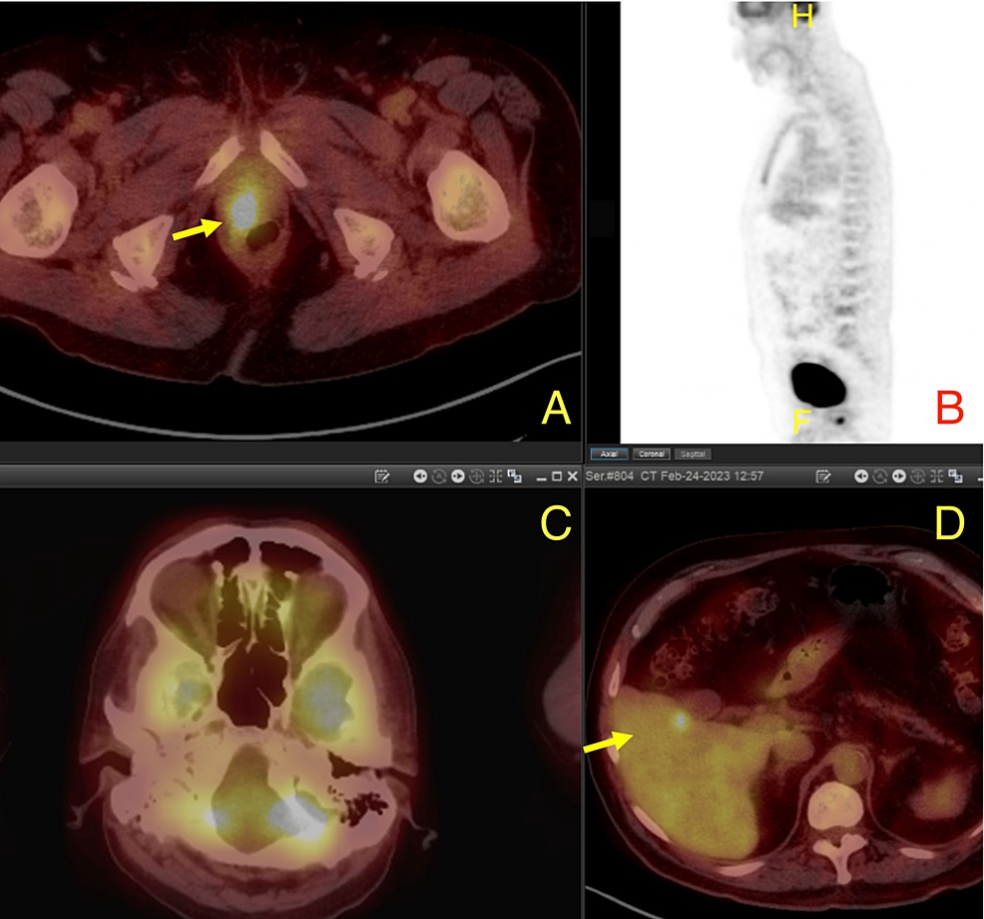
FDG-PET CT. (A) Increased uptake seen in apical-lateral prostate similar to area showing small cell neuroendocrine growth. (B) Skull not demonstrating enhanced growth near the area of orbital metastasis. (C) Spine not showing significant FDG enhancement. (D) Liver metastasis demonstrating increased uptake to FDG. FDG-PET: 18 F-fluorodeoxyglucose-positron emission tomography.
